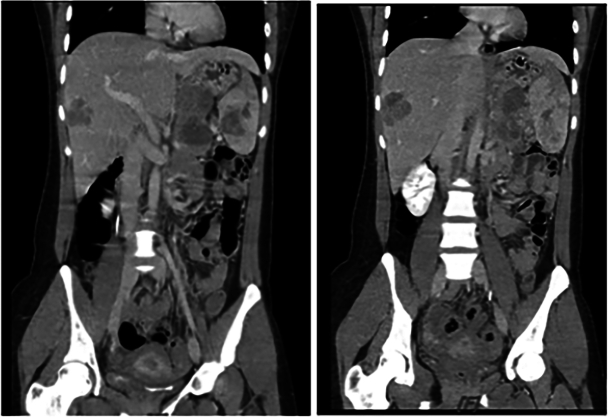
Pancreatic tail tumor with splenic vein and portal vein thrombosis, multiple liver and spleen metastasis, and ascites (abdomen computed tomography: coronal view).
